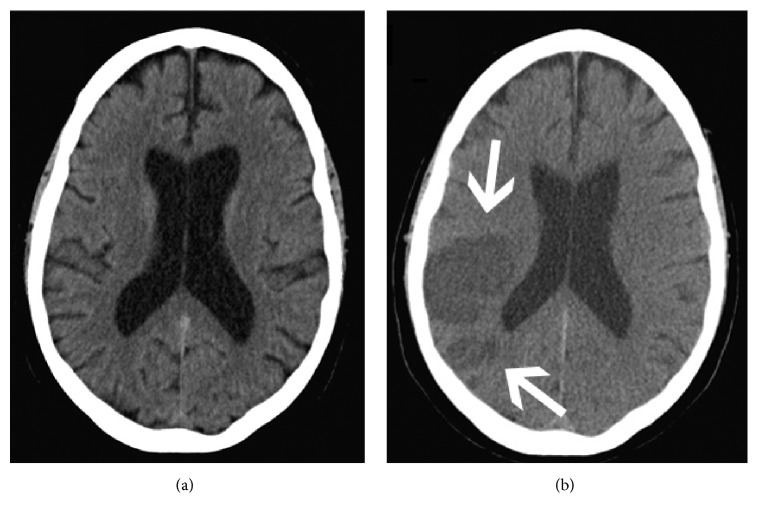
(a) Head CT at time of admission showing no acute findings. (b) Subsequent head CT showing acute multiembolic stroke (arrows).
